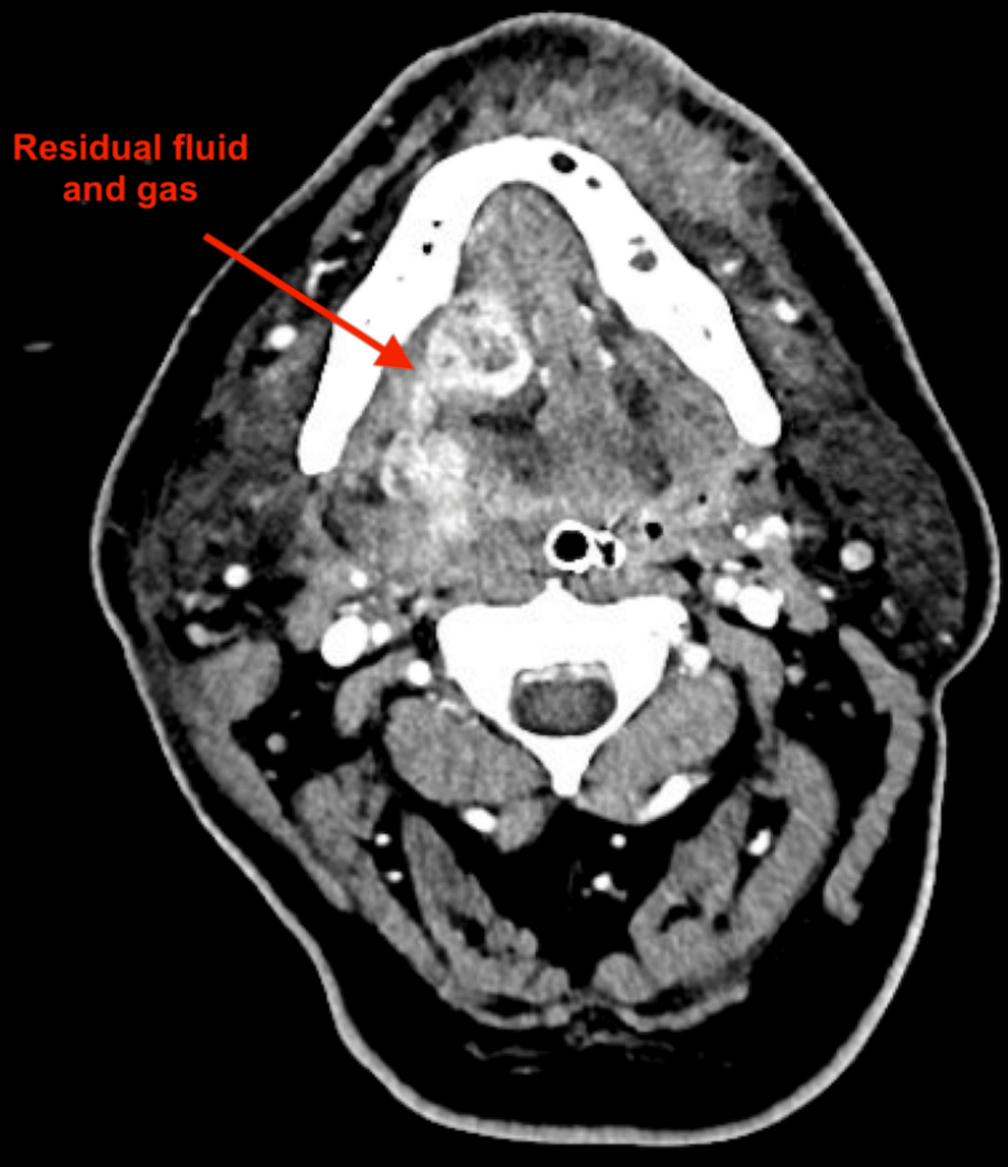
Post-operative CT soft tissue neck Interval surgical changes from drainage and debridement with residual gas and fluid collection (red arrow) medial to the right facial artery.
